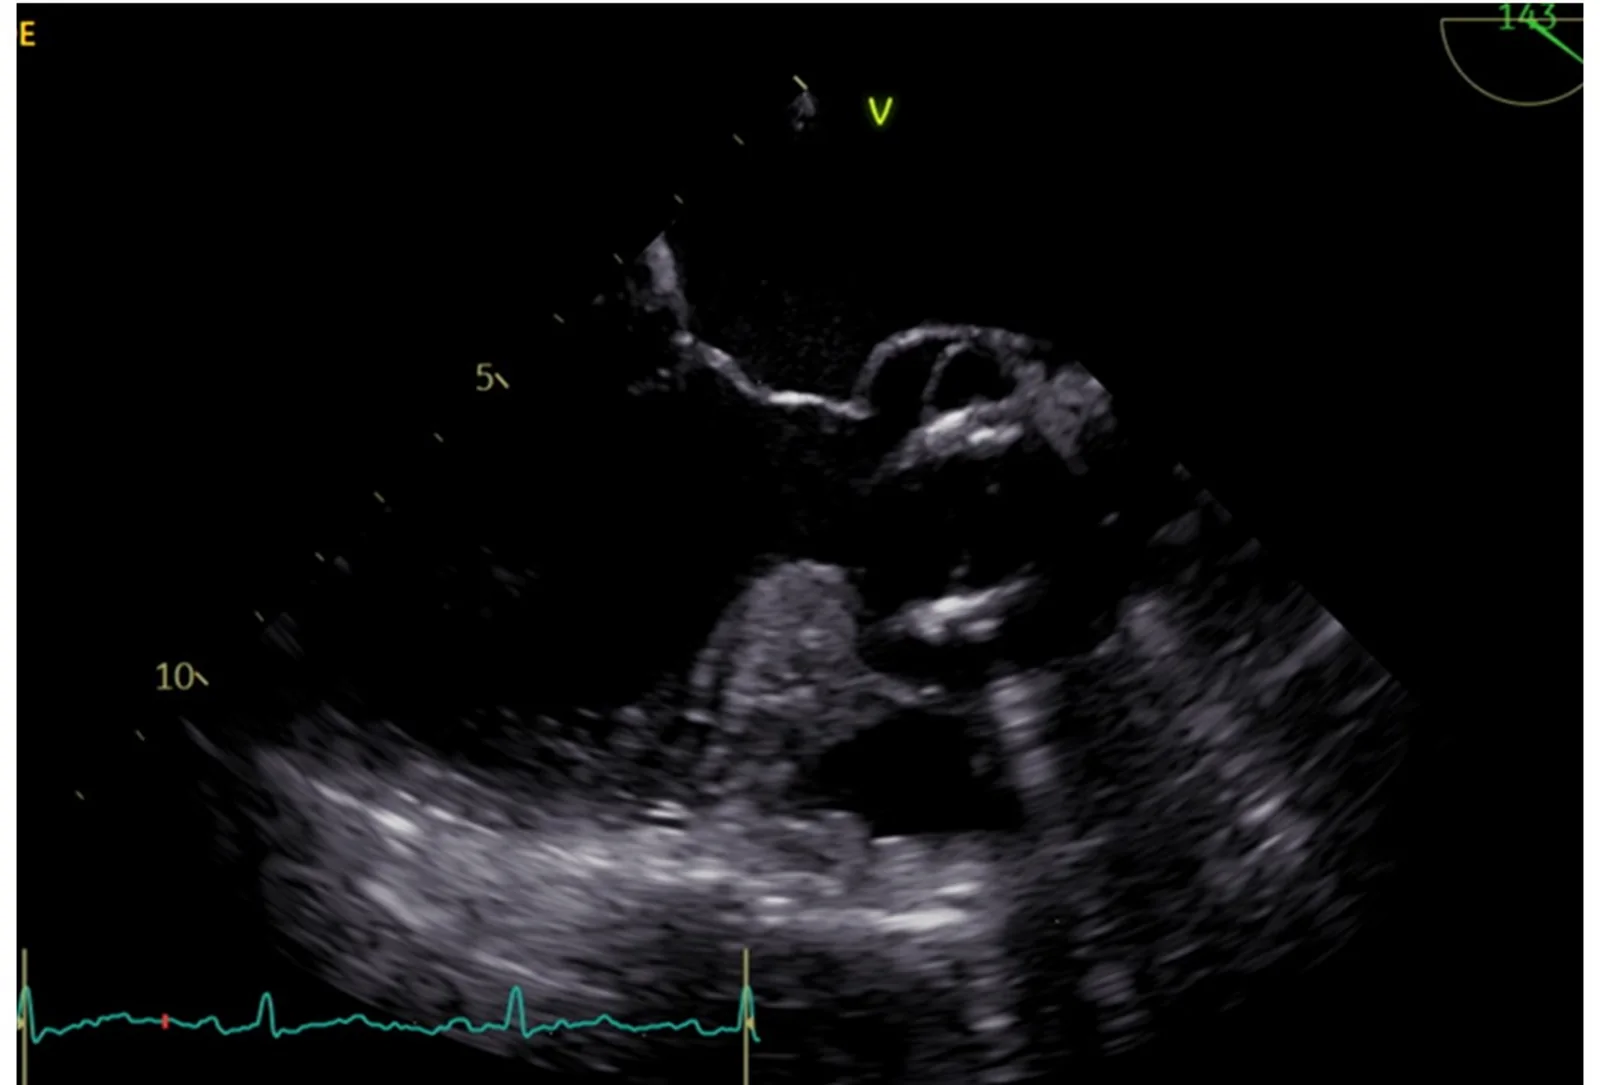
Preoperative transesophageal echocardiography. Preoperative transesophageal echocardiography showing marked rocking motion of the prosthetic aortic valve, suggesting prosthetic valve dehiscence and annular disruption
